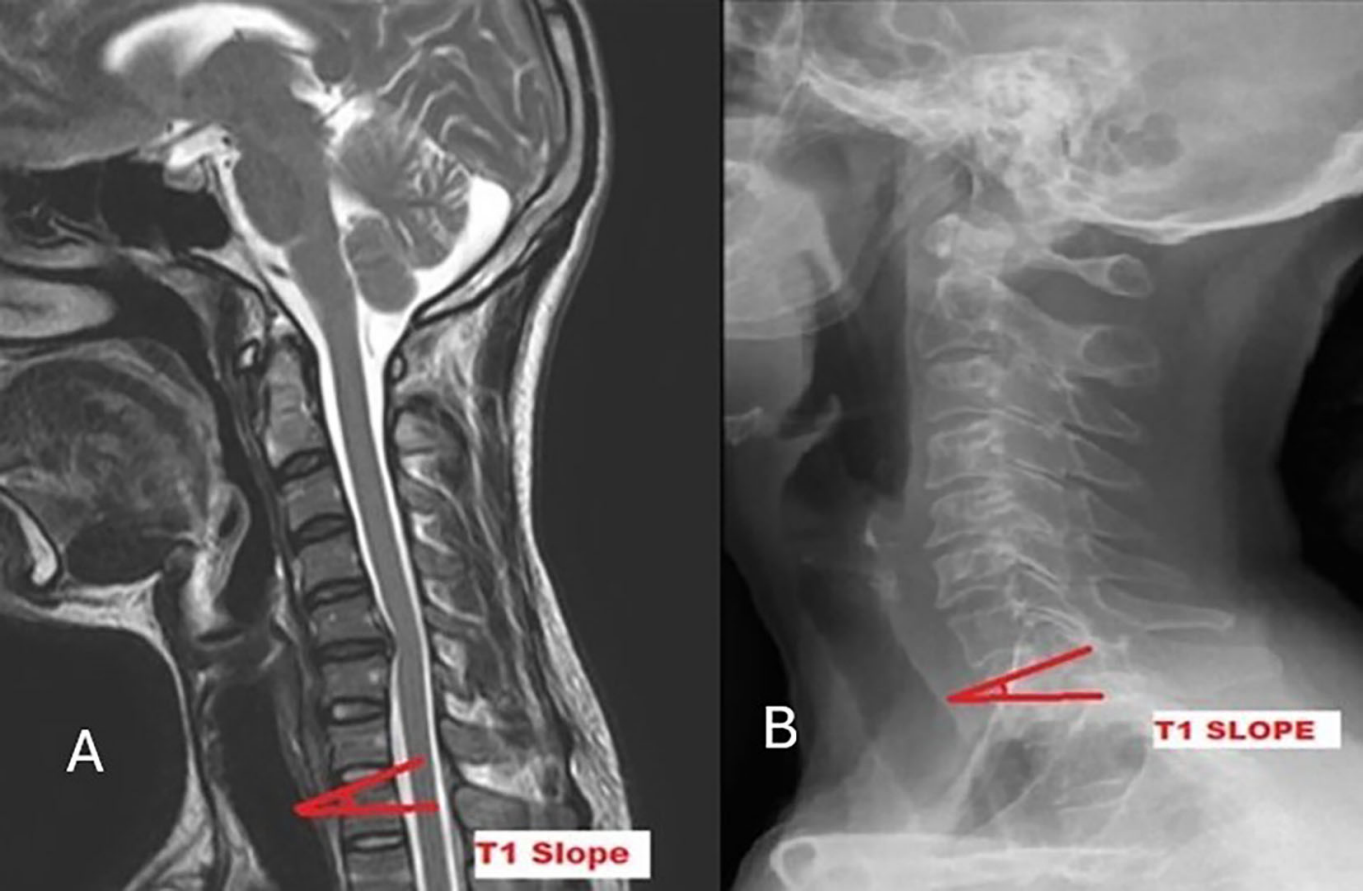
Measurement of T1 slope on MRI (A) and radiograph (B).
